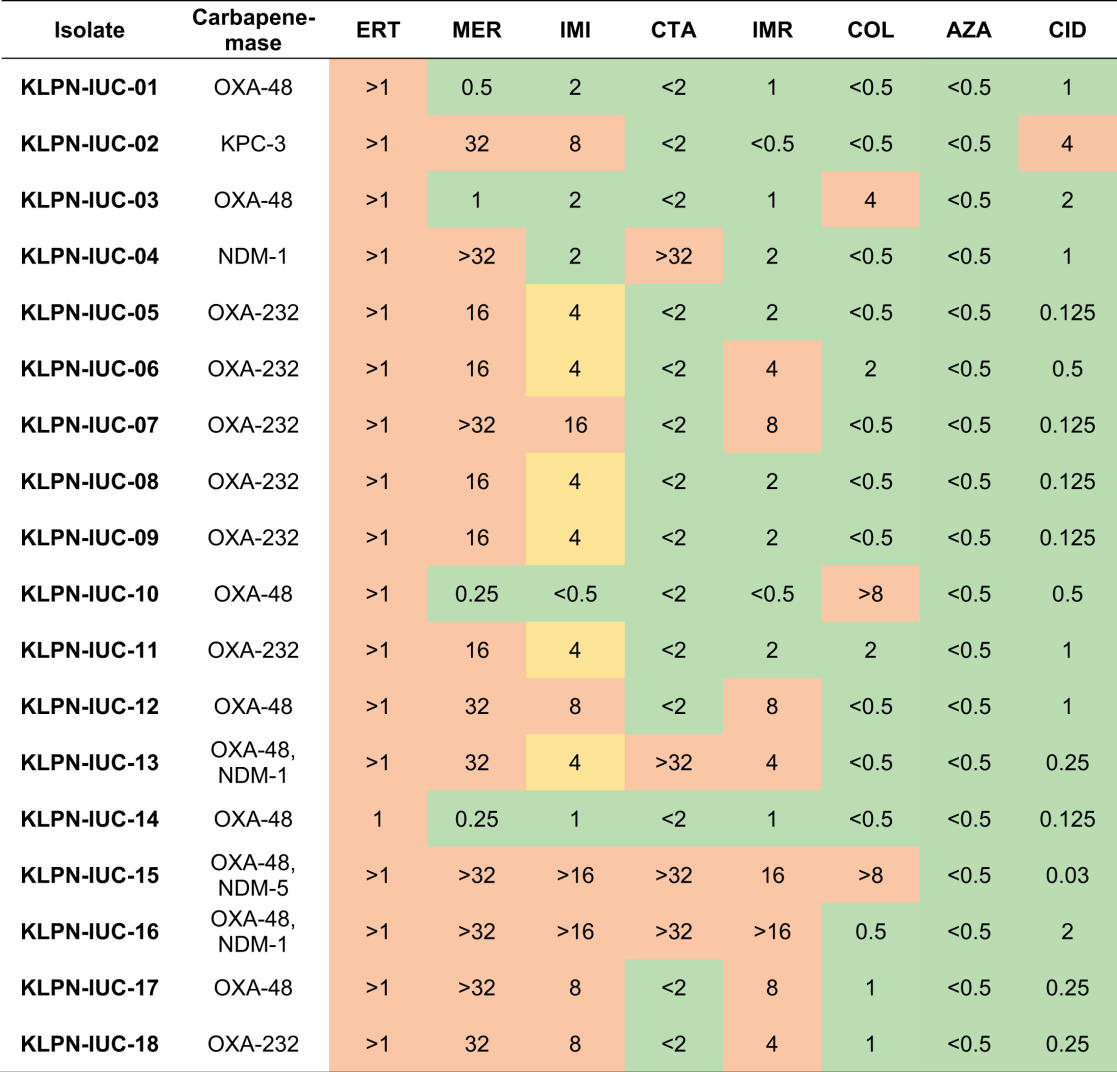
Minimal inhibitory concentrations (MICs) for last-resort antibiotics of *iuc*-positive carbapenemase-producing *K. pneumoniae* isolates from clinical samples at the University Hospital Cologne, Germany, 2013–2021. Interpretation of MICs according to EUCAST breakpoints is colour encoded; red = resistant; yellow = susceptible, increased exposure; green = susceptible, standard dosing regimen. For aztreonam-avibactam, breakpoints for aztreonam were applied. AZA, aztreonam-avibactam; CID, cefiderocol; COL, colistin; CTA, ceftazidime-avibactam; ERT, ertapenem; IMI, imipenem; IMR, imipenem-relebactam; MER, meropenem.
